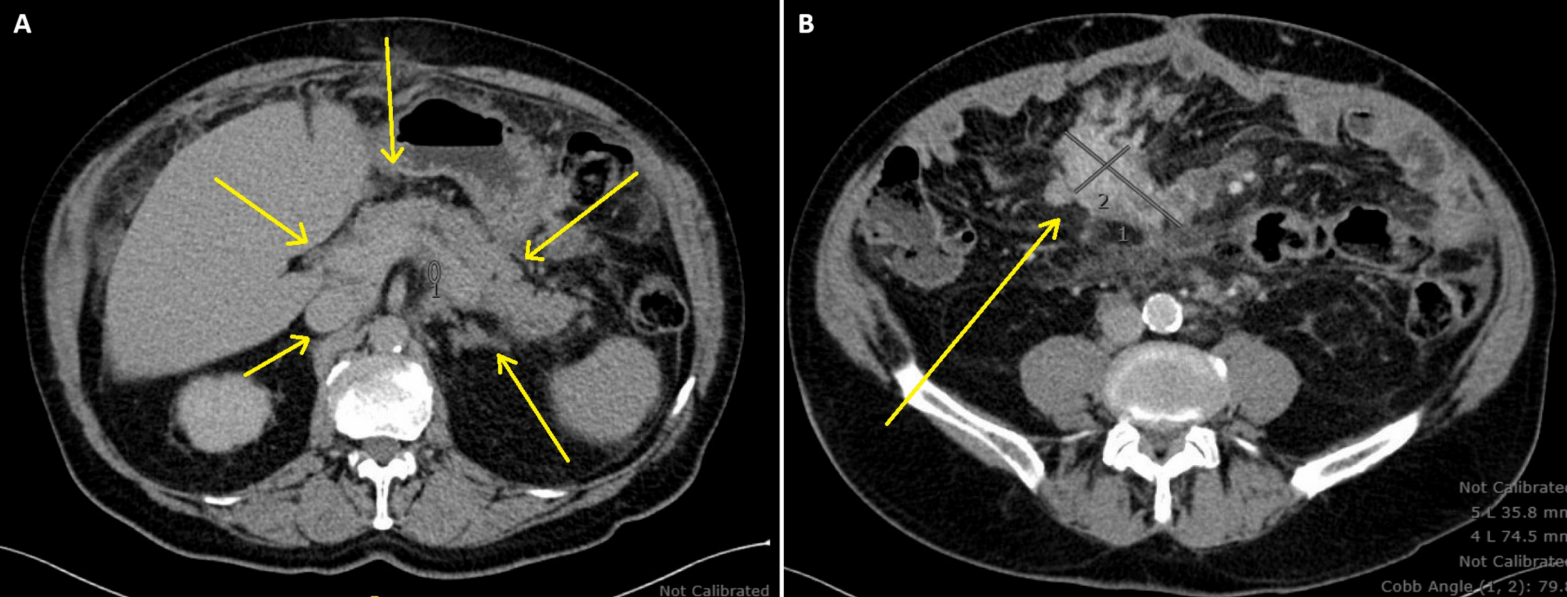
Abdominal computed tomography (CT). (A) Homogeneously enhancing, lobulated pancreatic parenchyma (yellow arrows). (B) Enhancing, spiculated soft-tissue lesion in the right central mesentery, suggestive of sclerosing mesenteritis (yellow arrow).
